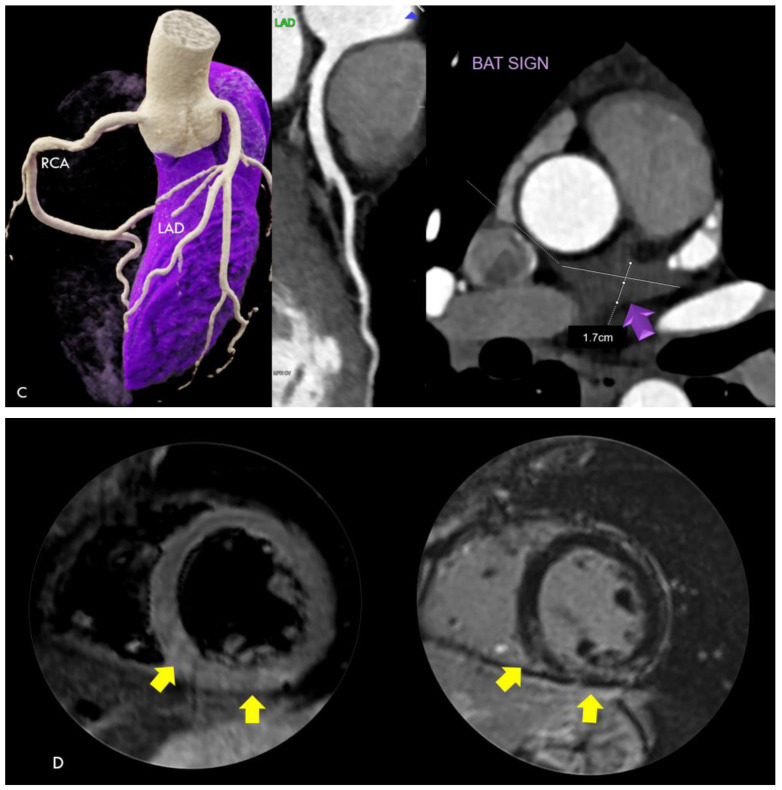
(A) Coronary CTA findings: Non-calcified hypodense lesion with low CT attenuation and 40% area stenosis in the mid right coronary artery (RCA) (arrow). (left)*:* Three-dimensional Volume Rendering Technique (3D-VRT). (right): Curved multiplanar reformations (cMPR). (B) The non-calcified lesion had low CT attenuation (86 HU) (left). Lesion-specific PCAT was positive (right) for perivascular edema (−21 HU), measured by a region of interest (ROI). (C) Left anterior descending (LAD), right coronary artery (RCA), and all other coronary arteries were normal ((left)*:* 3D-VRT and (mid): cMPR). Calcium score was zero. Prominent pericardial fluid (“bat sign”) within the superior epicardial recess *(*right). (D) Cardiac MRI showed myocardial edema (left) on turbo inversion recovery magnitude (TIRM) imaging in the midventricular basal inferior region (with maximal 54 ms relaxation time on T2-mapping), and focal late enhancement (right) (arrows) during the delayed phase.
